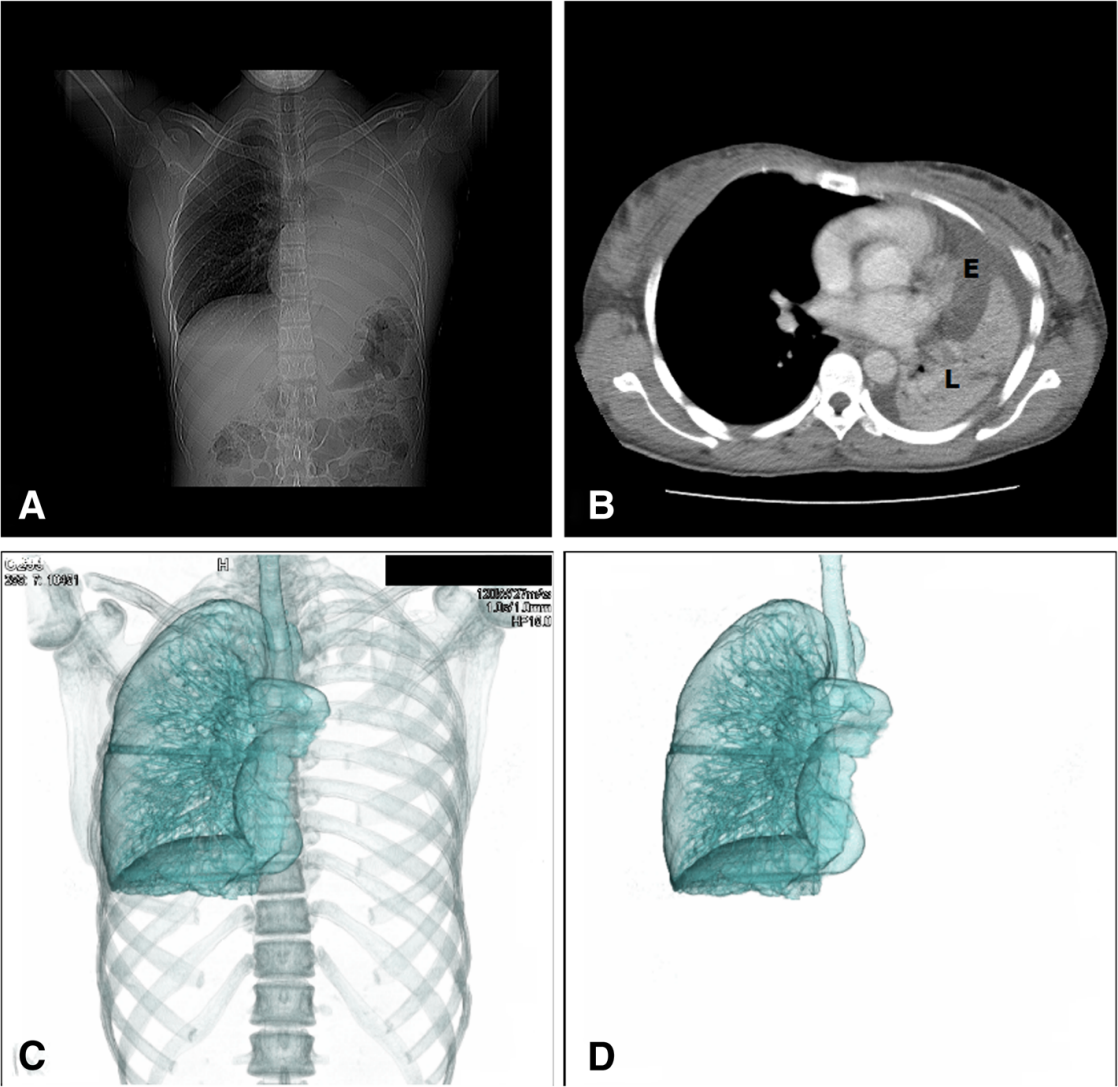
Findings detected with computed tomography. Panel A. Coronal CT of the chest showing left-sided lung collapse. Panel B. Axial CT of the chest revealing a pleural effusion (E) and the collapsed left lung (L). There is left mediastinal shift; the right lung is hyper-inflated. Panel C. Three dimensional (3D) reconstruction with volume rendering depicting complete collapse of the left lung with an obstructed left main bronchus. Panel D. Skeletal system removed from the 3D reconstruction.
